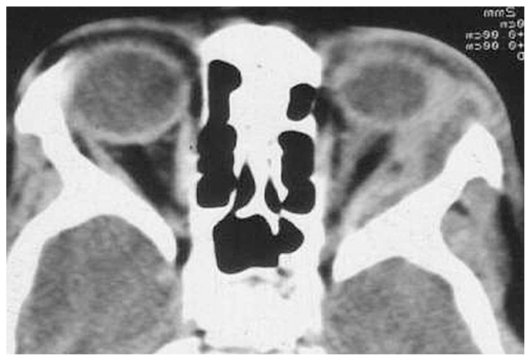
Contrast-enhanced computed tomography depicting a ring-enhancing, low density lesion in the lateral extraconal space of the left orbit. Note medial displacement of the globe and lateral rectus.
